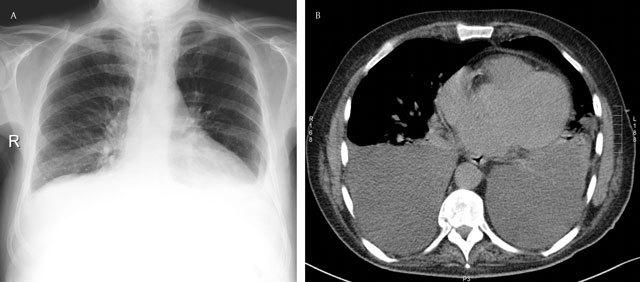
Subpulmonary effusion – Frontal chest radiograph (a) shows an elevation of the left and right hemidiaphragm (“pseudo-diaphragm). There is an obliteration of the intrapulmonary blood vessels. Note the blunting of both costophrenic sulcus. The gastric air bubble is not seen. Corresponding axial CT image (b).
